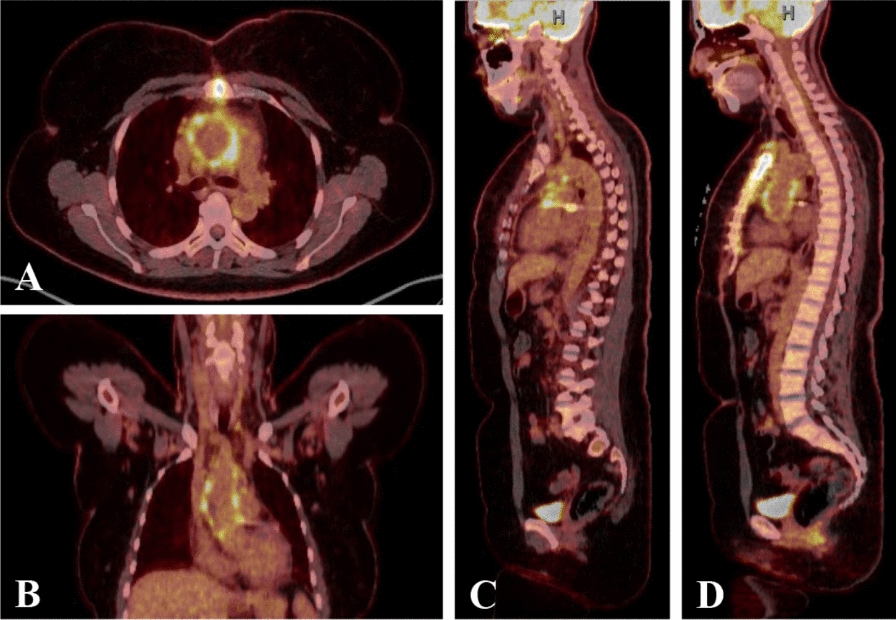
Postoperative FDG-PET CT of the chest. A, B Hemashield platinum graft replacement of ascending aorta, with circumferential intense activity (SUVmax = 13.7) consistent with graft material. B, C Sagittal full body views showing the absence of aortic inflammation throughout the remaining portions of the aorta down to the aortic bifurcation. Abbreviations: *CT* computed-tomography, *FDG* fluorodeoxyglucose, *PET* positron emission tomography, *SUV* standardized uptake value
